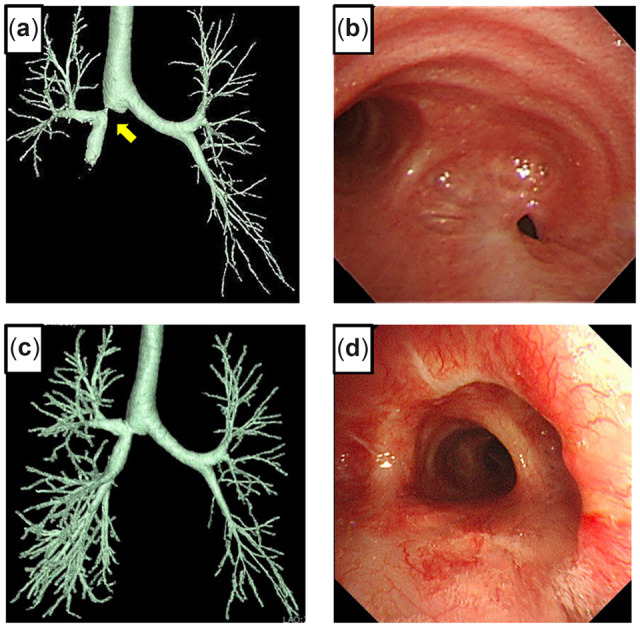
Three-dimensional computed tomography shows severe stricture (arrow points to the stricture) (a). Bronchoscopy shows a pinhole with granulated tissue at the orifice of the right main bronchus (b). A 3-dimensional scan shows decent passage of the anastomosis (c). Bronchoscopy 3 months after surgery shows healing and lumen patency of the anastomosis (d).
